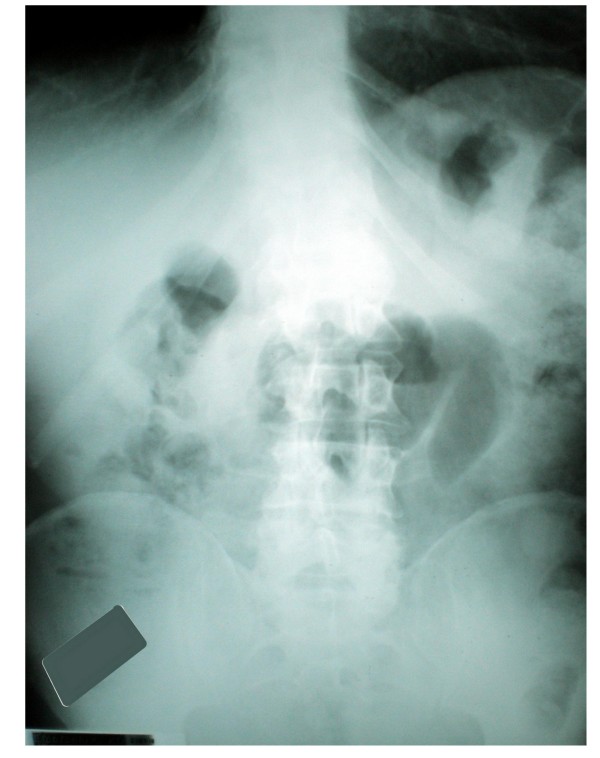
Plain abdominal radiograph showing dilated loops of small bowel in the right hemiabdomen and a soft tissue mass.
